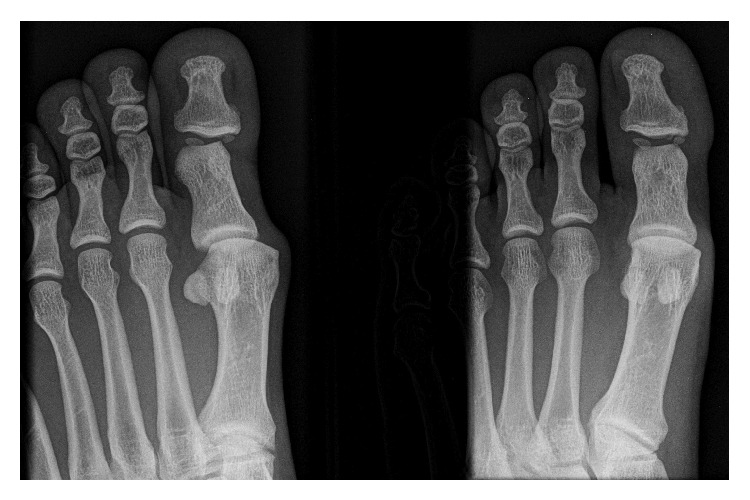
This is the dorsoposterior and oblique radiographs obtained at the emergency department. It reveals an oblique IP joint space of the left great toe, raising suspicion of a dislocation. As a true lateral radiograph was not obtained, the diagnosis was missed.
